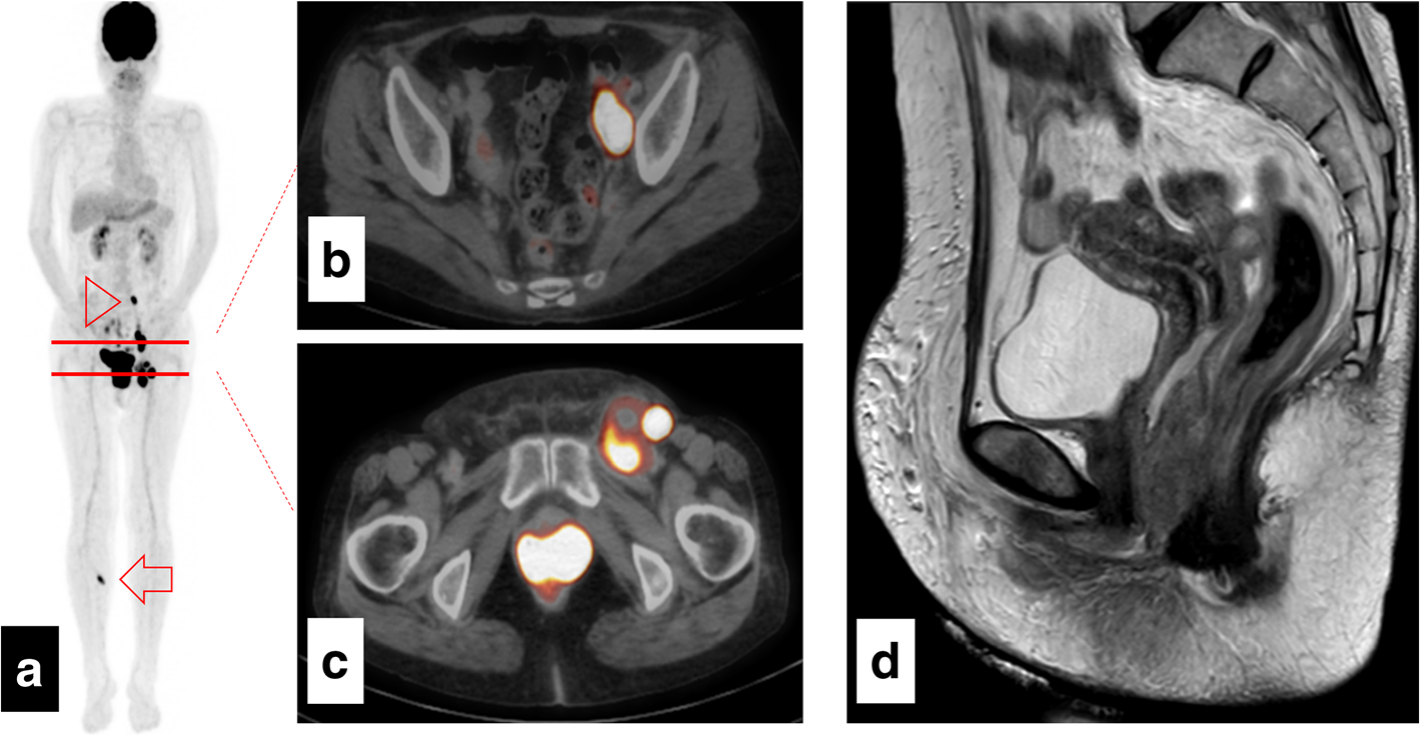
Images from ^18^F-fluoro-deoxyglucose positron emission tomography–computed tomography performed 5 days after the initiation of itraconazole (itraconazole) treatment (a, b, c). A magnetic resonance image acquired 12 days after the initiation of itraconazole treatment (d). a The arrowhead shows paraaortic lymph node metastases, and the arrow shows metastases to the right foot. b Pelvic lymph node metastases. c Left inguinal lymph node metastases. d Thickening of the lower two-thirds of the posterior vaginal wall
